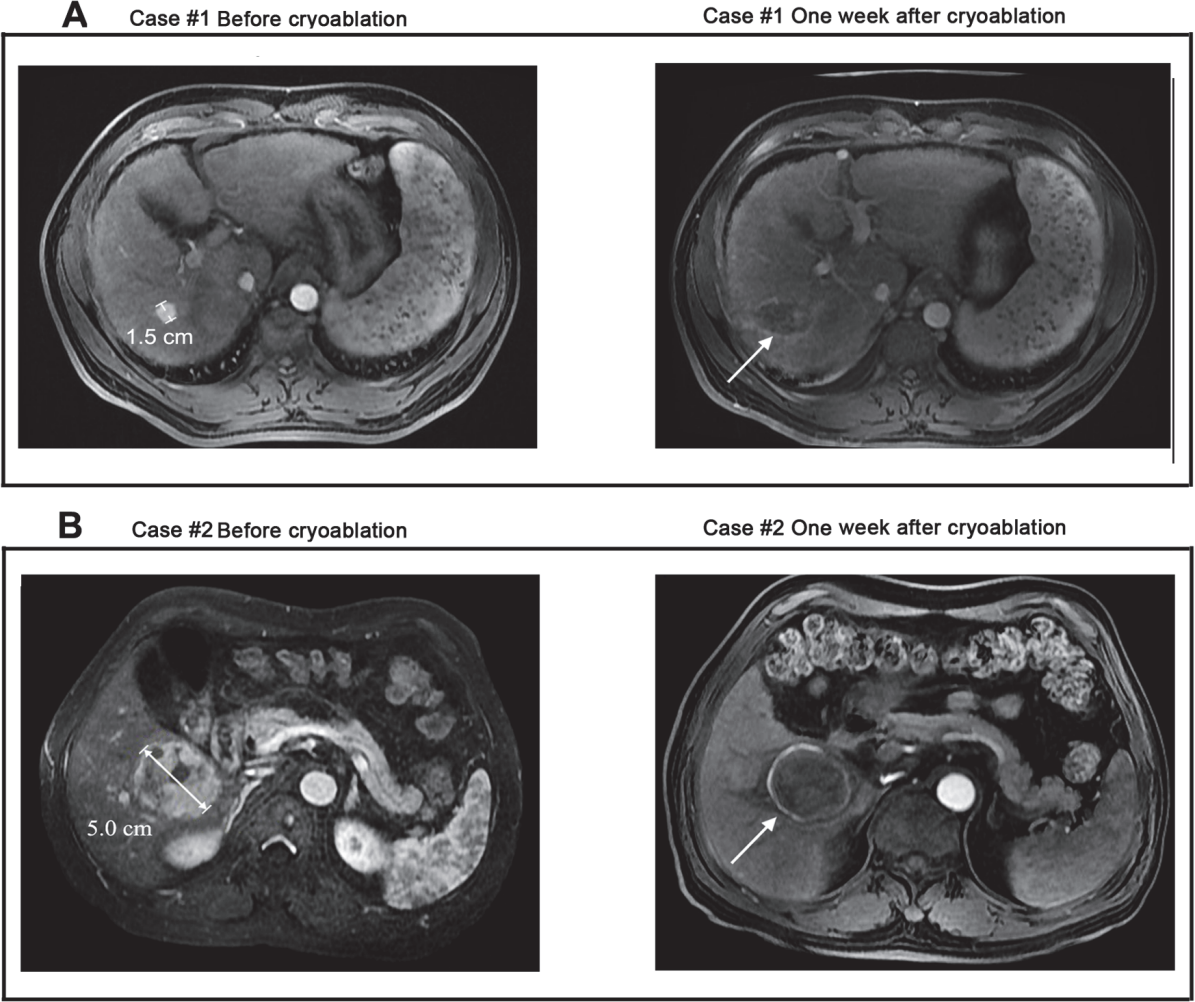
MRI images of 2 representative cases achieved CR from cryoablation. A. Case #1, a 50-year-old male with a single HCC nodule of 1.5 cm that was completely cryoablated. At the end of follow-up, he was still alive with tumor free for 120 months after treatment. B. Case #2, a 48-year-old female with a single HCC nodule of 5.0 cm that was completely cryoablated. At the end of follow-up, she was still alive with tumor free for >108 months after treatment.
